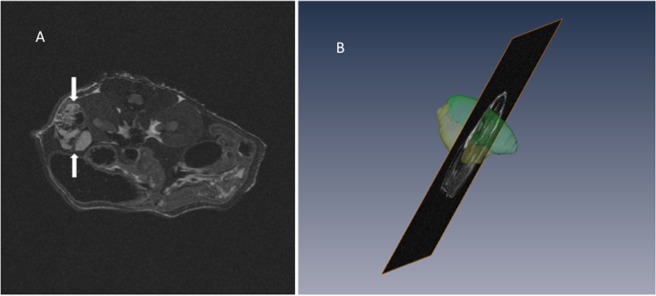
(A) Example of axial MRI T2W-image. The right and left kidneys are clearly visible with a contrasting tumor on the left kidney (between the arrows). The high signal intensity in the tumor reflects the presence of increased fluid in the tissues. (B) Example of 3D reconstruction of tumor (yellow) on the left kidney (green) using AMIRA software.
